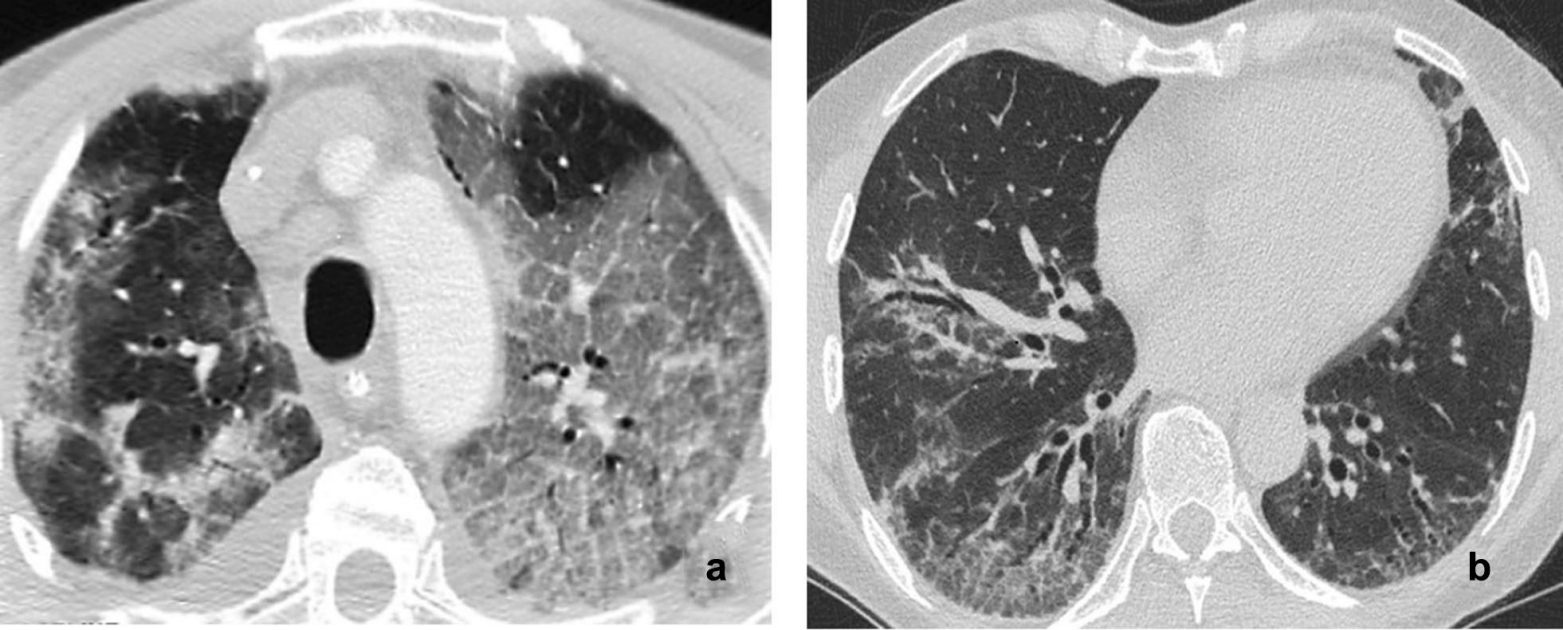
a Contrast-enhanced computed tomography scan of the thorax of Patient 5 at the level of the carina at ICU admission (slice thickness 2 mm). The picture shows bilateral, diffuse ground-glass opacities with partial subpleural sparing and crazy-paving pattern. b Follow-up high-resolution computed tomography scan of the thorax of Patient 4 at the level of the middle lobe (slice thickness 1 mm). The picture shows bilateral, mainly dorsal ground-glass opacities with reticulations and traction bronchiectasis
